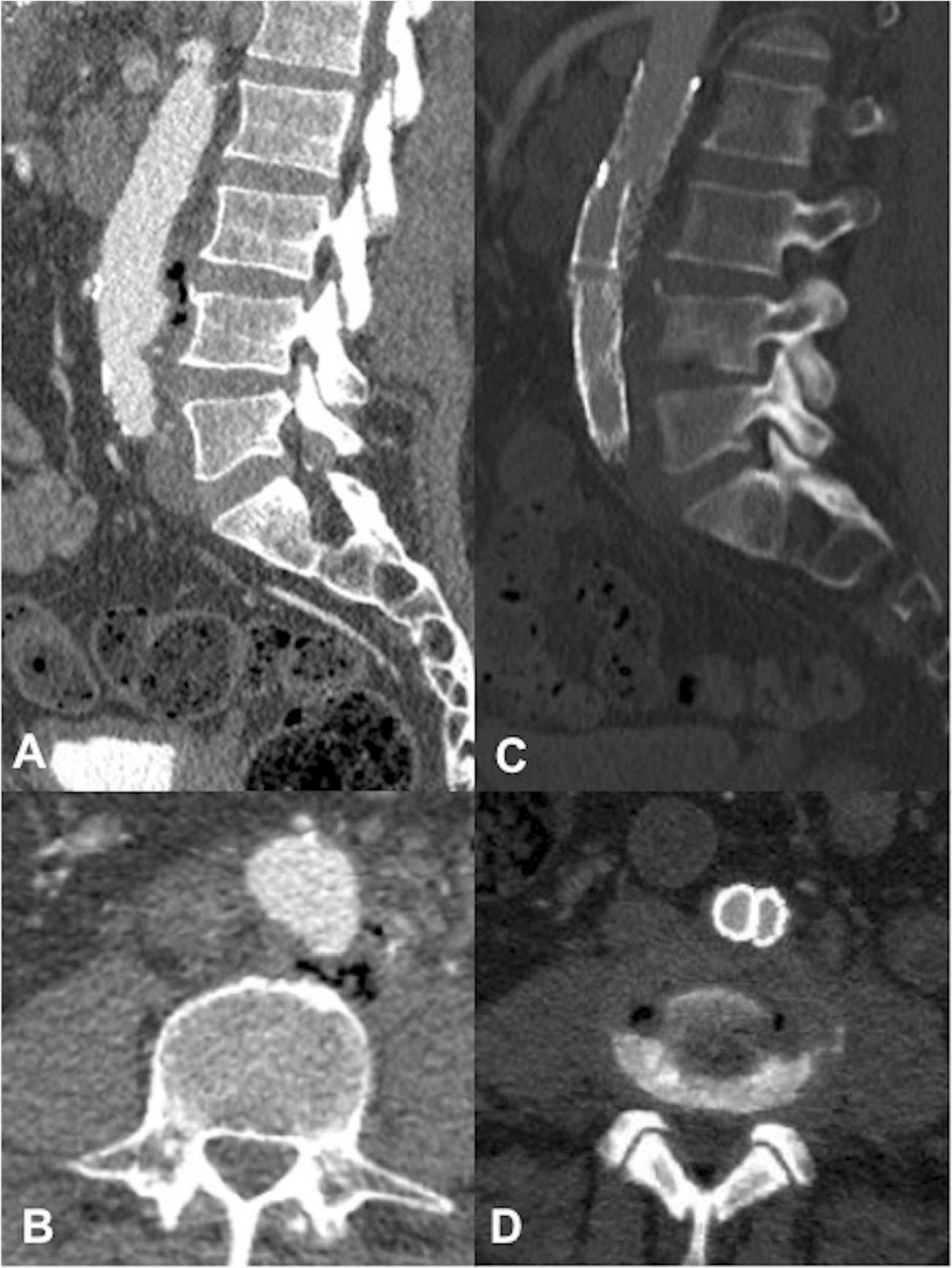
A 58-year-old man (patient 5). a Sagittal and b axial CT angiogram pre-EVAR. There is saccular aneurysmal dilatation at the L3/4 level with adjacent gas locules typical of an infective aneurysm. *E. coli* bacteraemia confirmed the infective aneurysm. c Sagittal and d axial images from routine surveillance CT 2 months post-EVAR demonstrate new L4/5 endplate erosion, anterior L4 vertebral body erosion, gas locules in the disc space and paravertebral soft-tissue density extending to the stent graft
